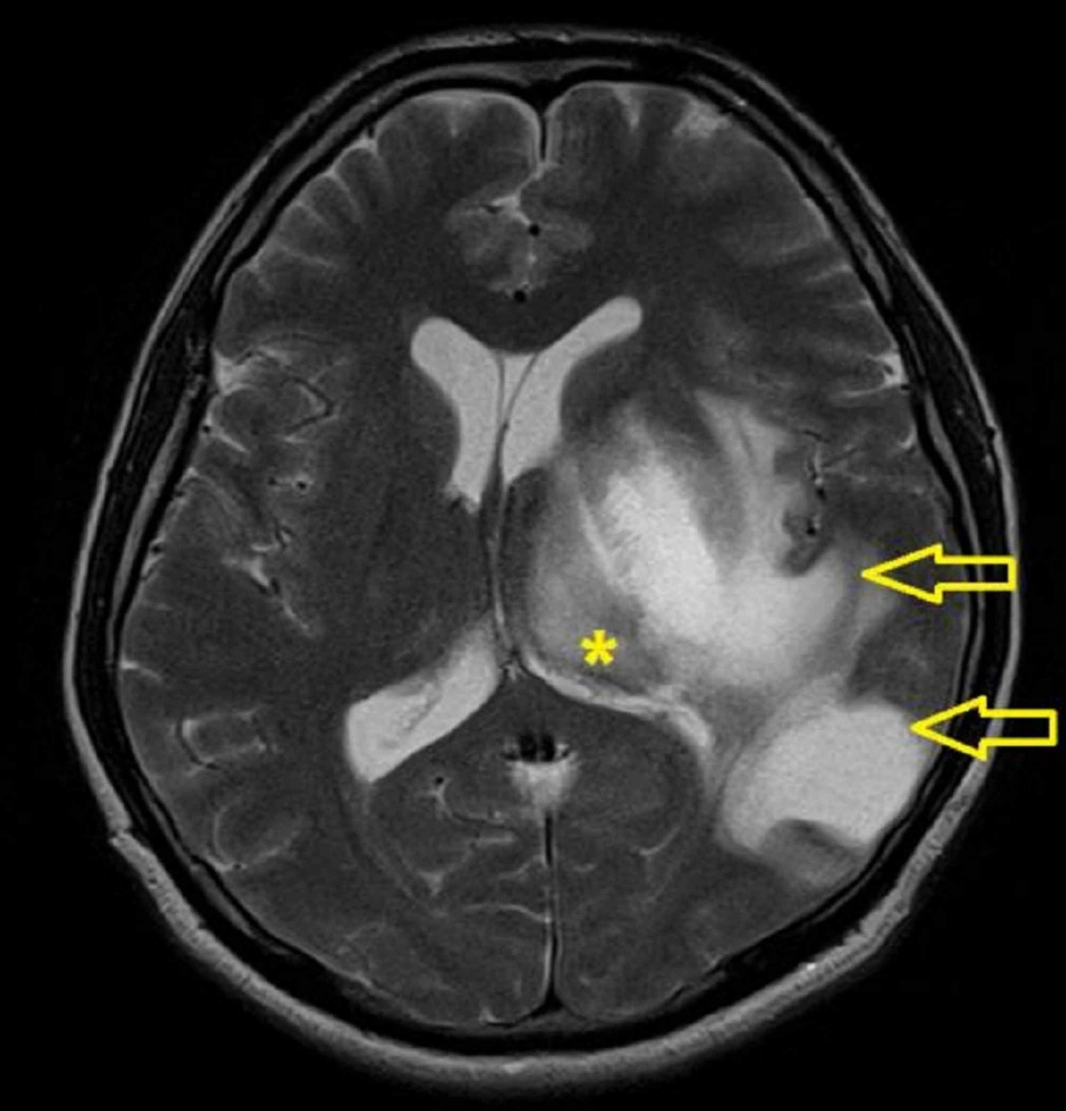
Magnetic resonance imaging (MRI) of the brain showed increased intensity within the left thalamus (*) and the left temporoparietal region (open arrow)
